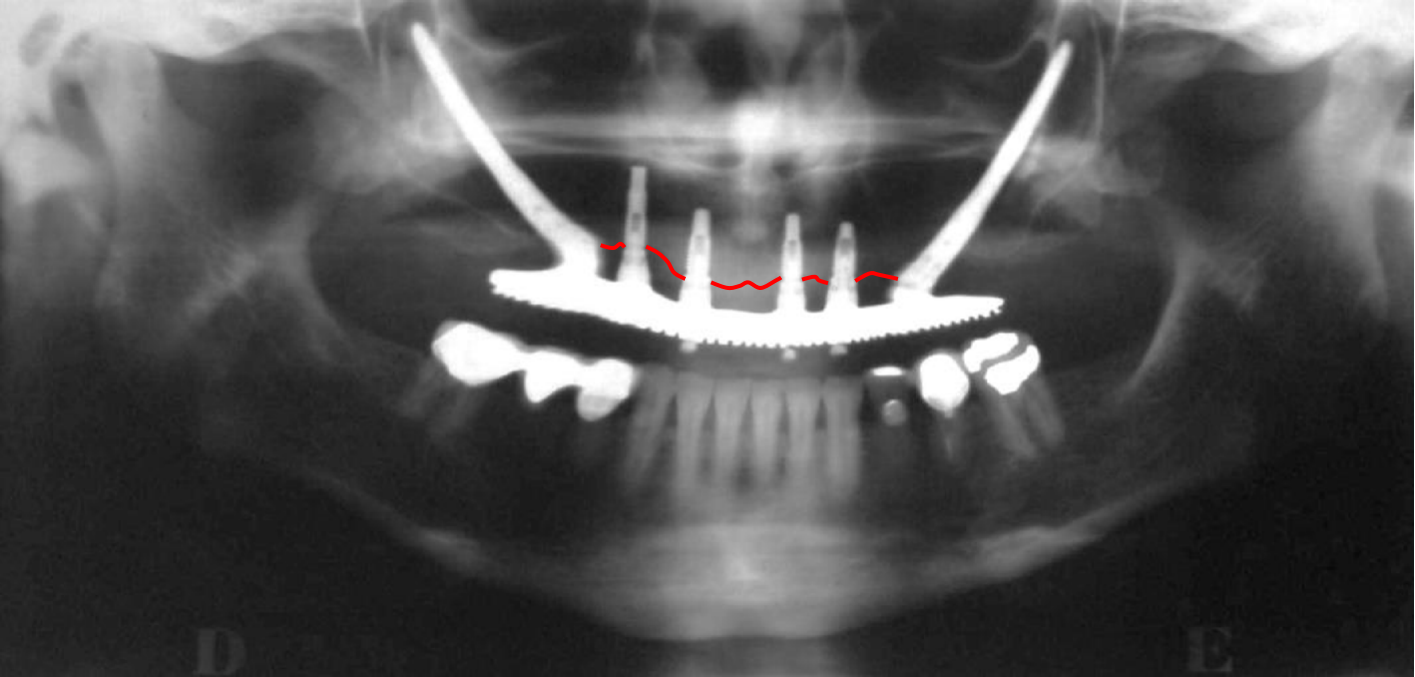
Panoramic radiograph showing bone level maintenance around the conventional implants
